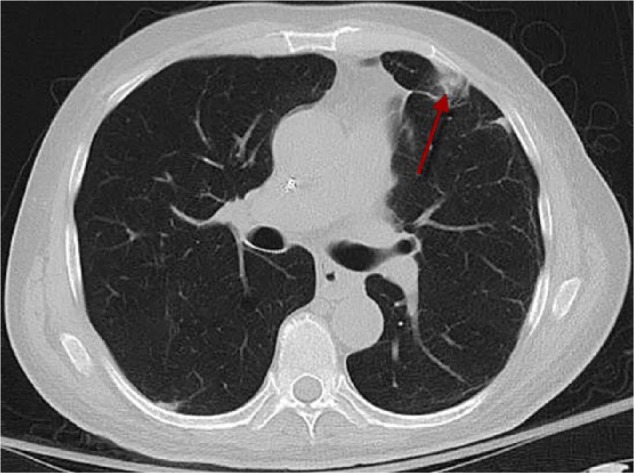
Repeat computed tomography scan of the chest after rifampin, isoniazid, pyrazinamide, and ethambutol therapy showing significant improvement in the size of the mass.
